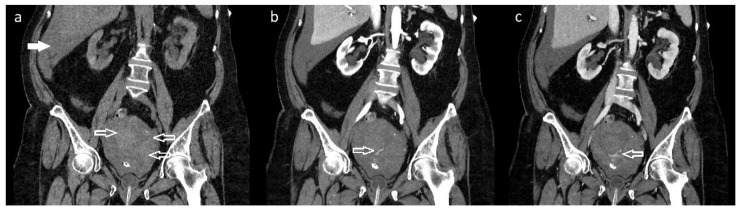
Abdominal computed tomography (CT) scan with the application of 90 mL intravenous contrast medium Visipaque 320 revealed a uterine tumor involving the cervix (size: 40 × 50 × 70 mm), hematometra with active bleeding from the tumorous mass, and a hemoperitoneum. (a) Non-contrast: coronal plane with hematometra in the enlarged uterus with tumor (arrows) and hemoperitoneum located in the Morison’s pouch (full arrow). (b) Contrast enhanced: coronal plane (arterial phase 30 s after applying the contrast agent) where an active extravasation of the contrast agent from the dorsocaudal part of the tumor can be seen (arrow). (c) Contrast enhanced: coronal plane (venous phase 70 s after applying the contrast agent). Continued process of the extravasation of the contrast agent, pointing to actively bleeding arterial area (arrow). A 67-year-old patient was referred to our department with a sudden onset of sharp lower abdominal pain and an episode of vaginal bleeding. The patient had a short history of intermittent, low-intensity postmenopausal bleeding and the diagnosis of pelvic mass on the ultrasound (although the diagnostic process for this was incomplete). Upon admission the patient had tachypnea, tachycardia, and diffuse abdominal rigidity. A speculum vaginal examination showed a small and extremely hard to visualize portion of ectocervix and, by bimanual palpation, a tumorous mass (involving both cervix and parametria) was diagnosed (minimal vaginal bleeding present). An urgent computed tomography scan was ordered which revealed an enlarged uterus with a tumor in the cervical region and active extravasation in the arterial and venous phase after applying the contrast, which pointed to active arterial bleeding from the lesion causing hematometra and subsequently uterine rupture and hemoperitoneum. We considered an acute surgical intervention as an unfavorable option because it was a locally advanced disease which had altered the pelvic anatomy in an already hemodynamically unstable patient. There was also a significant drop in hemoglobin levels (123 g/L vs. 100 g/L samples taken in approximately 1.5 h interval), which pointed to a major blood loss. We were informed about the possibility of a UAE and, after the required initial management and blood transfusions, the patient was transported to the angiography department.
